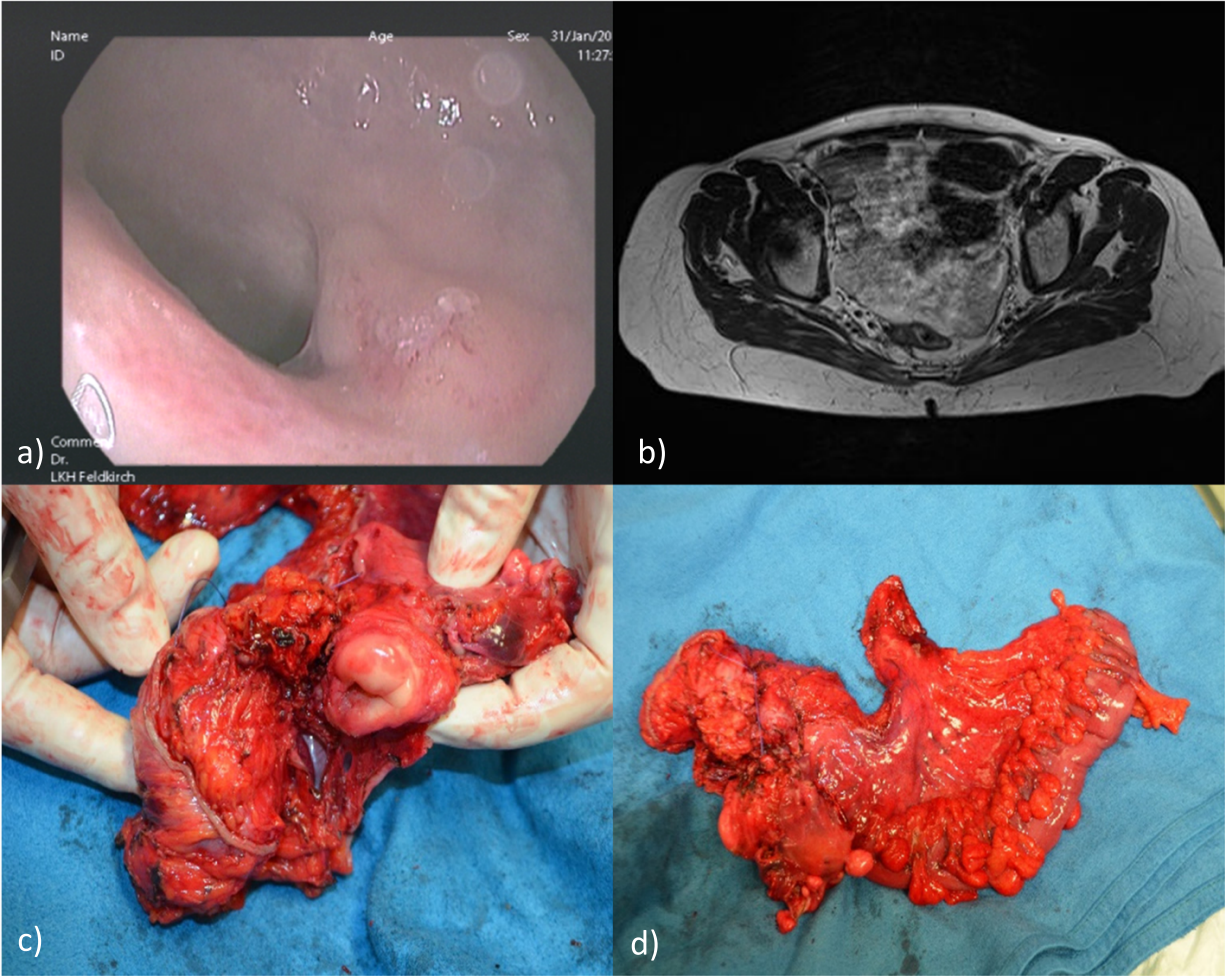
a, b Endoscopy after chemoradiation therapy with a good clinical and imaging (magnetic resonance imaging) response. On the assumption of a malignant transformed teratoma of the ovary an open total mesorectal excision and hysterectomy en bloc was performed as operative strategy (c) and (d)
